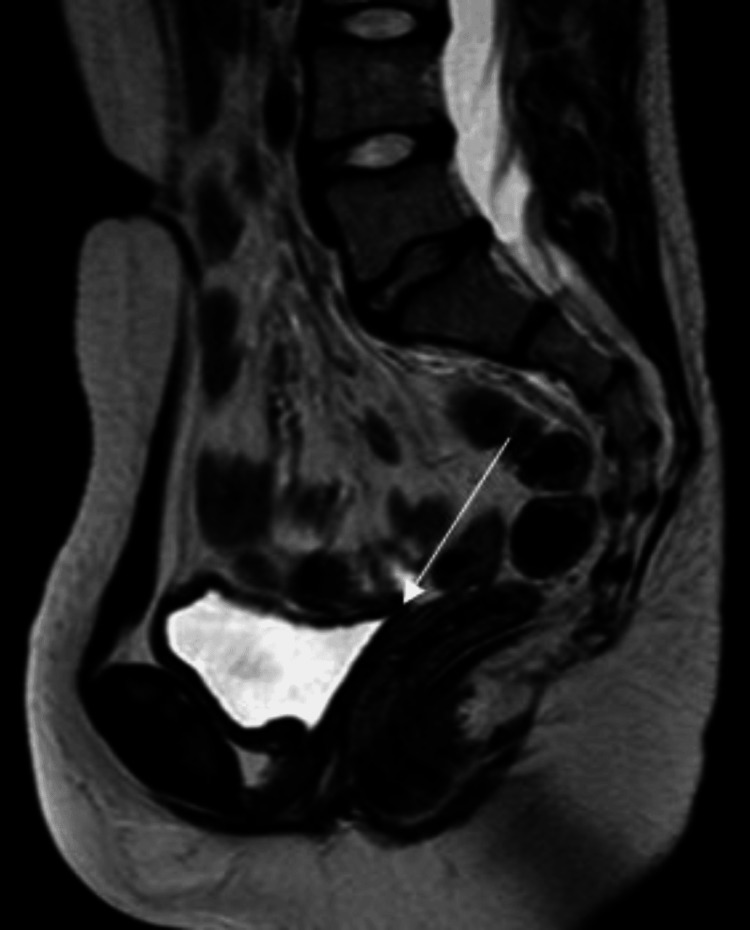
T2-weighted MRI of the pelvis. The sagittal view reveals the absence of the uterus, cervix, and the upper two-thirds of the vagina in the index case (the arrow points to the empty rectovesical pouch).
